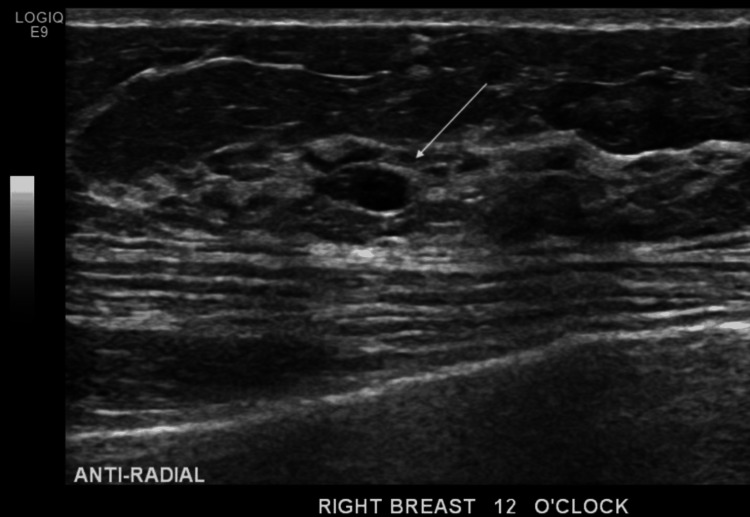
Ultrasound of the right breast showing a fibroadenoma (arrow)
